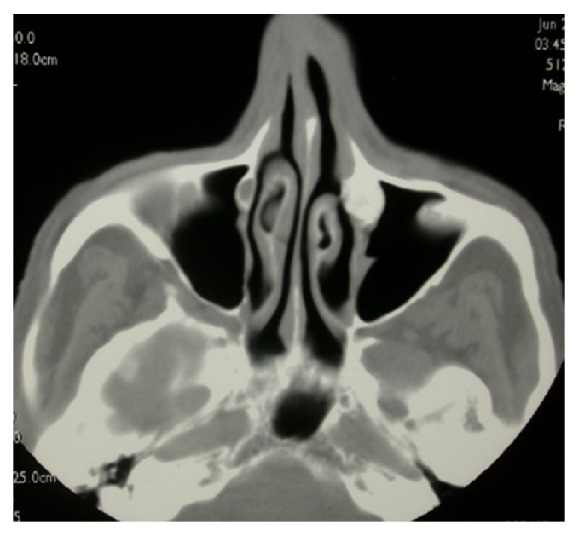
Axial view of the CT scan of the paranasal sinus showing the osteoma of the medial wall of the left-sided maxillary sinus.
